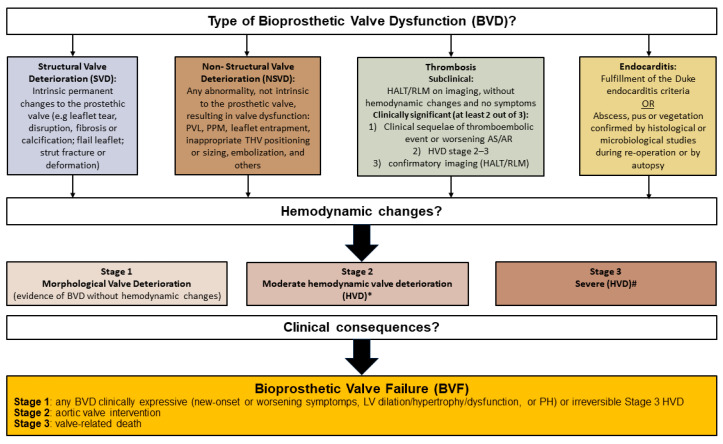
Classification of bioprosthetic valve dysfunction (BVD) and bioprosthetic valve failure (BVF) [30]. * Moderate HVD: Increase in mean trans-valvular gradient ≥ 10 mmHg resulting in mean gradient ≥20 mmHg with concomitant decrease in EOA ≥0.3 cm^2^ or ≥25% and/or decrease in Doppler velocity index ≥0.1 or ≥20% compared with echocardiographic assessment performed 1–3 months post-procedure, OR new occurrence or increase of ≥1 grade of intra-prosthetic AR resulting in ≥ moderate AR. # Severe HVD: Increase in mean transvalvular gradient ≥20 mmHg resulting in mean gradient ≥30 mmHg with concomitant decrease in EOA ≥0.6 cm^2^ or ≥50% and/or decrease in Doppler velocity index ≥0.2 or ≥40% compare with echocardiographic assessment performed 1–3 months post-procedure, OR new occurrence, or increase of ≥2 grades of intra prosthetic AR resulting in severe AR. AR: aortic regurgitation; AS: aortic stenosis; HALT: hypo-attenuated leaflet thickening; LV: left ventricle; PH: pulmonary hypertension; RLM: reduced leaflet motion.
